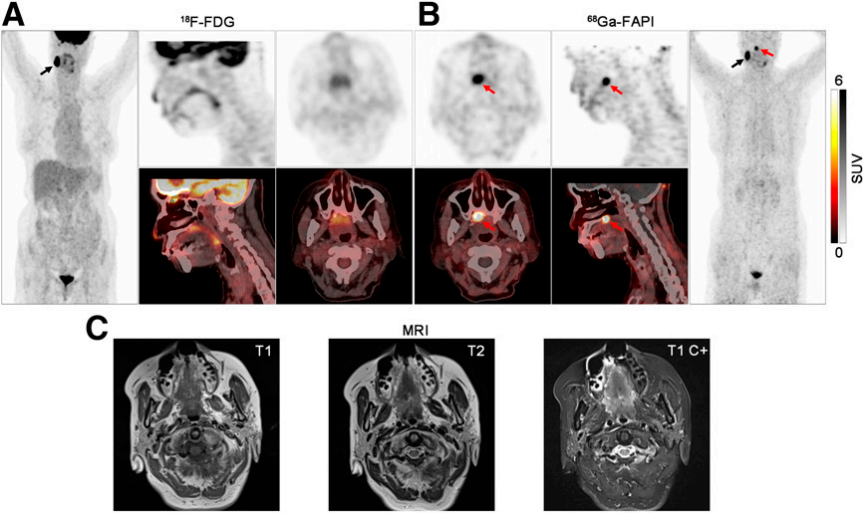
PET/CT and MR images of 72-y-old woman (patient 30) pathologically confirmed with metastatic squamous cell carcinoma of right neck. (A) ^18^F-FDG PET images (left and top) and PET/CT images (bottom), shown in coronal, sagittal, and axial views (from left to right), demonstrated metastatic lymph node of right neck with intensive metabolic activity (black arrow, SUV_max_, 30.1) but presented no evidence for primary tumor. (B) ^68^Ga-FAPI PET images (top and right) and PET/CT images (bottom), shown in axial, sagittal, and coronal views (from left to right), also detected metastatic lymph node with high ^68^Ga-FAPI activity (black arrow, SUV_max_, 16.3). There was intensive uptake of ^68^Ga-FAPI in palate (red arrow, SUV_max_, 11.3). (C) T1-weighted, T2-weighted, and contrast-enhanced T1-weighted MRI also presented no evidence for primary tumor. Subsequent surgery confirmed mucoepidermoid carcinoma of palate. C+ = contrast-enhanced.
